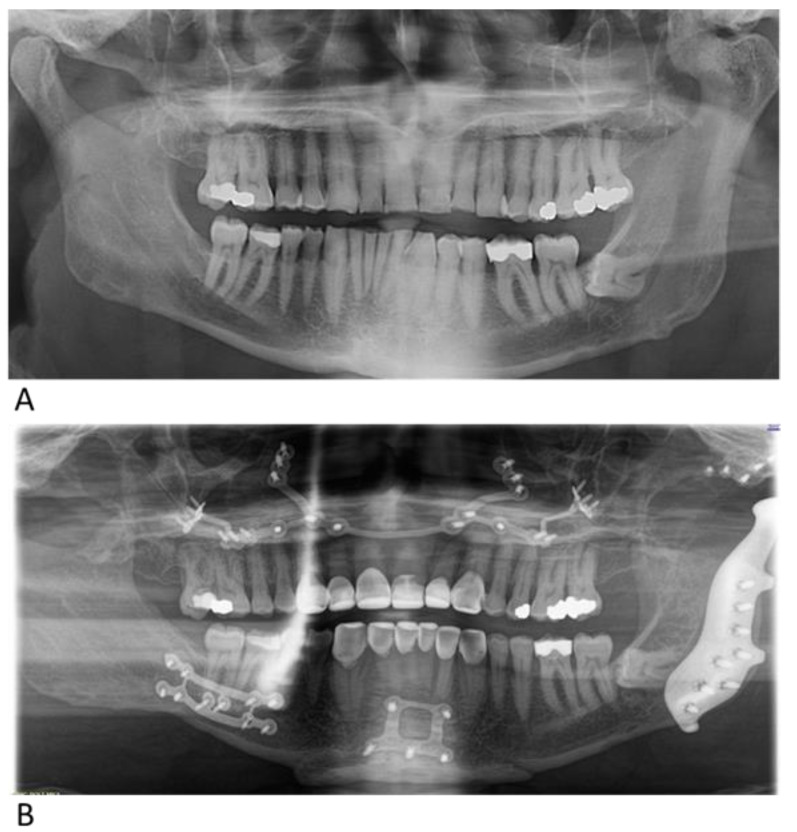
Preoperative (A) and postoperative (B) panoramic radiographs of case 3.
